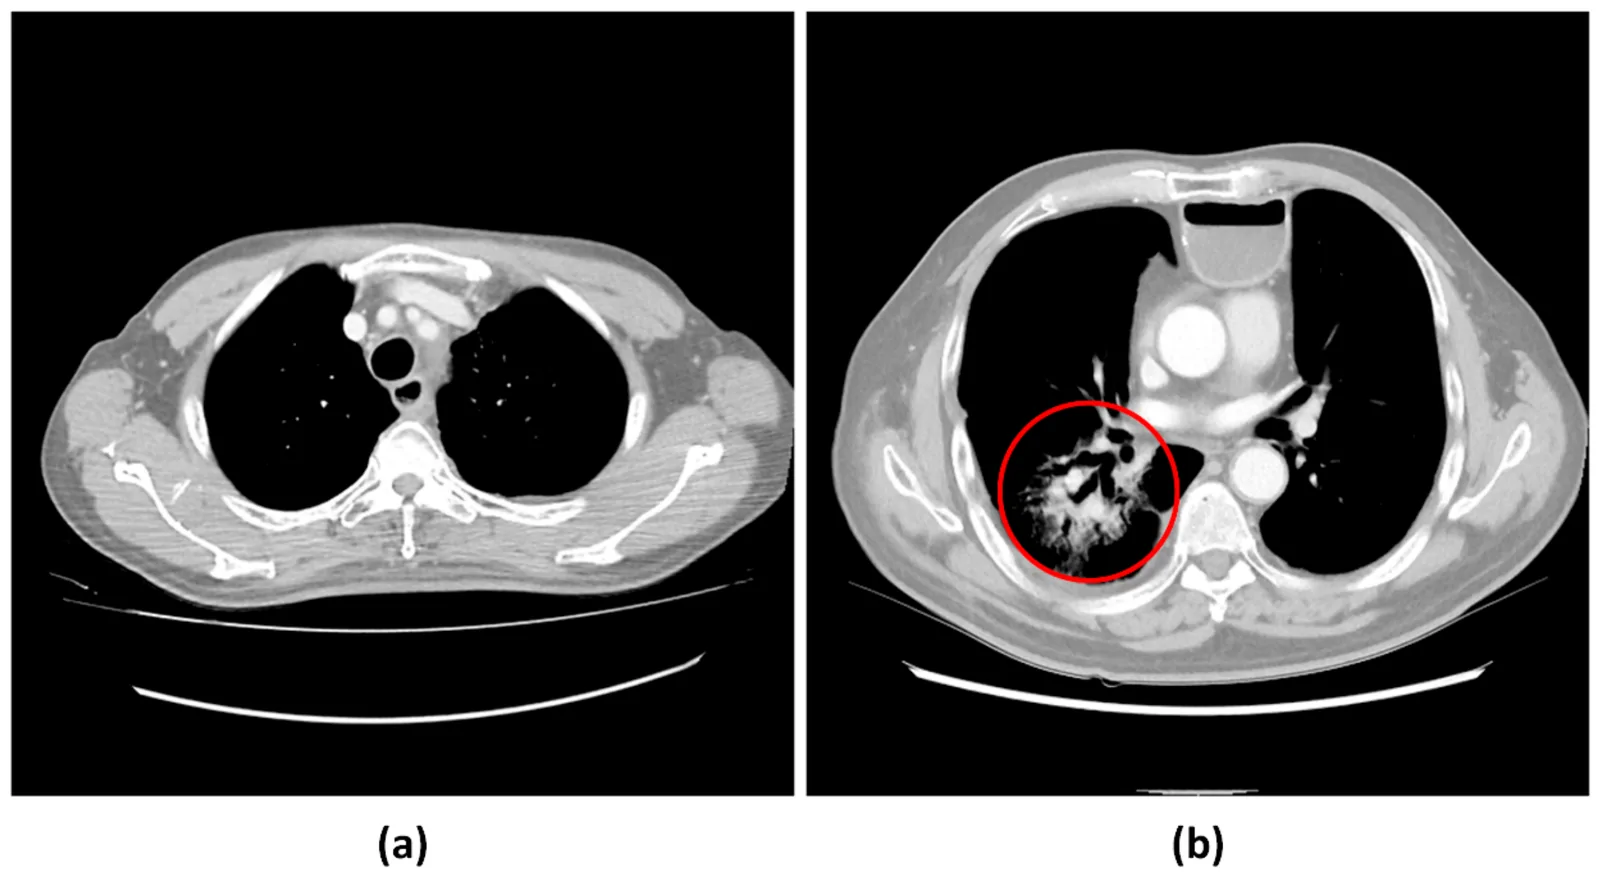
(a) Normal CT image and (b) RP CT image, with the red circle indicating a suspected RP-related region for qualitative visual reference. Abbreviations: CT: Computed Tomography, RP: Radiation Pneumonitis.
